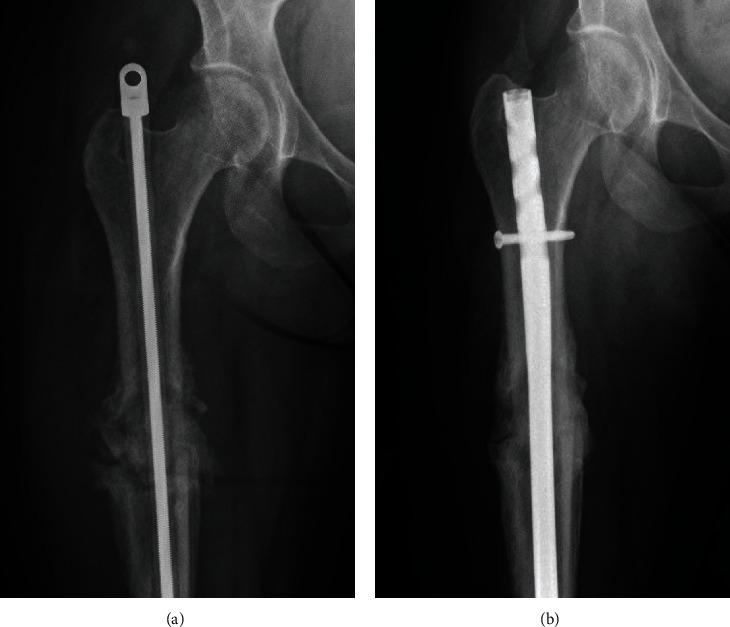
(a) Radiograph of right femur status post intramedullary fixation with an antibiotic-coated nail, approximately one month after fracture. There is significant increased bridging bone at the site of the prior pathologic fracture of the proximal right femoral shaft, consistent with fracture healing. (b) Radiograph of right femur status post intramedullary nail exchange and definitive fixation, approximately six months after fracture. Bony callus formation is evident in the region of the fracture with unchanged alignment.
